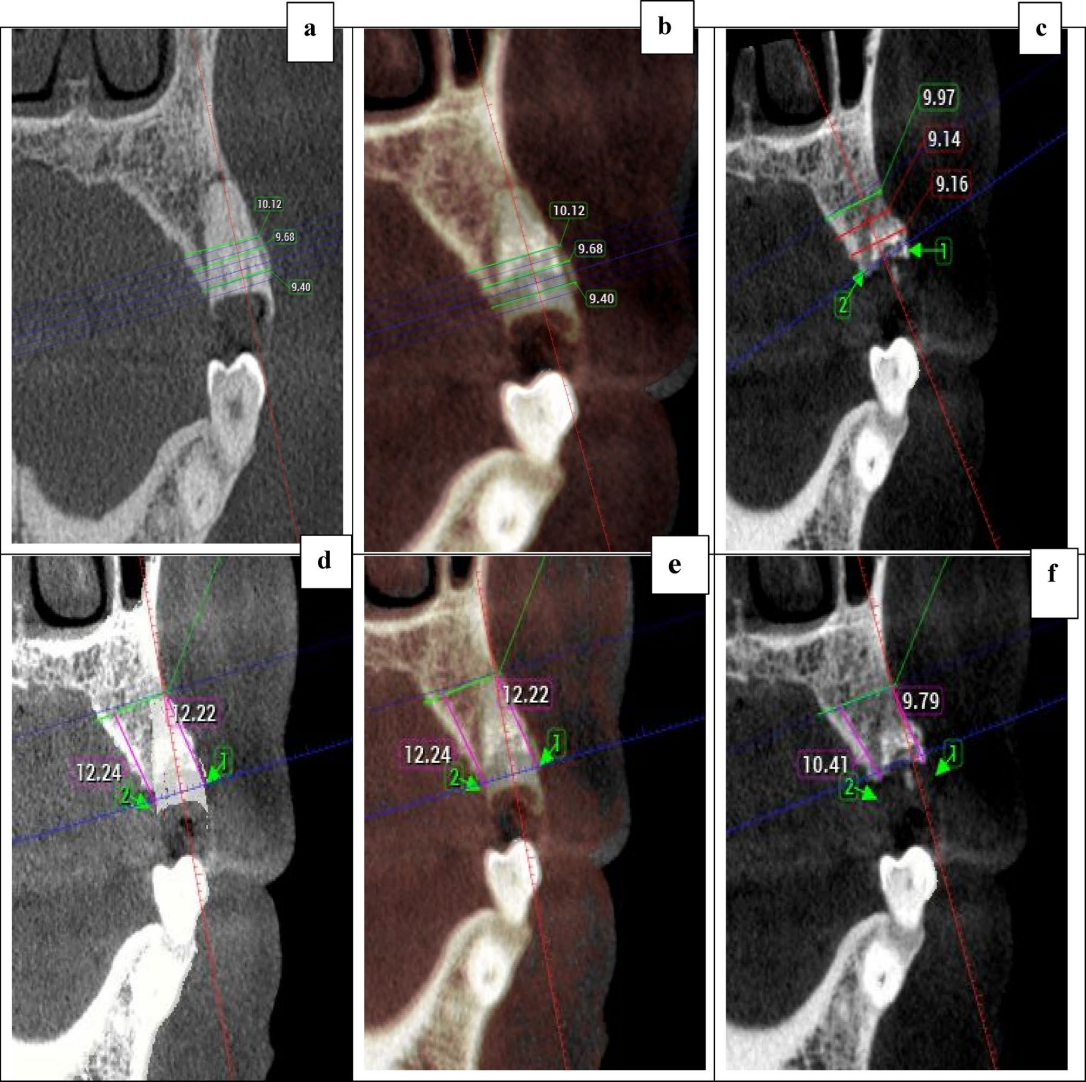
Cone-beam computed tomography (CBCT) evaluation., (a) initial CBCT scan with ridge width measurements at 1, 3 and 5 mm below the most coronal aspect of the crest, (b) fusion superimposition of the initial and 9 months post-operative scans, (c) 9 months postoperative scan, (d) initial CBCT scan showing a tangential line drawn at the most apical point of the socket depth parallel to the horizontal plane, points marked at the most crestal parts of the buccal and palatal ridges and line dropped to measure buccal and palatal ridge heights, (e) fusion superimposition of the initial and 9 months post-operative scans, (f) 9 months postoperative scan.
